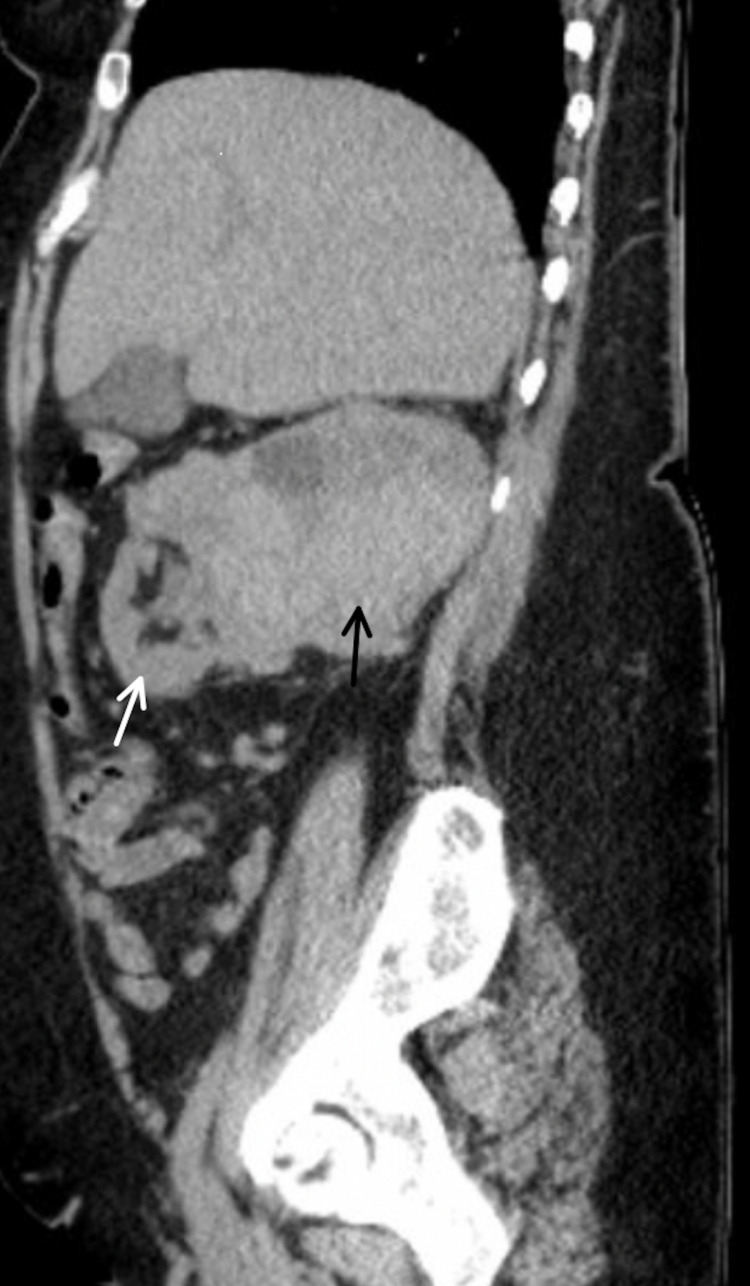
Sagittal View of the Right-sided Renal Mass Sagittal computed tomography (CT) image showing a large multilobulated 12.8 cm x 9.3 cm solid and cystic right-sided renal mass. The white arrow points towards the normal portion of the kidney. The black arrow points towards the solid and cystic renal mass.
